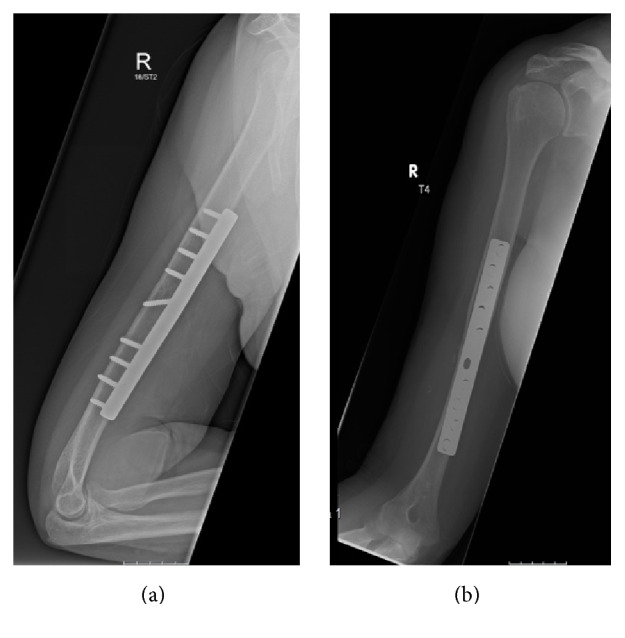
Radiographs demonstrating surgical fixation with a dynamic compression plate.
